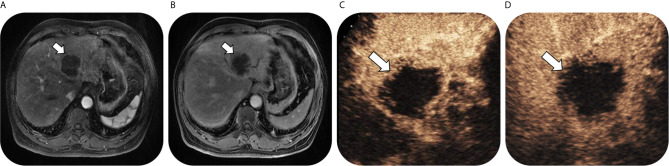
Images in a 63-year-old man with chronic hepatitis B virus infection and pathological confirmed intrahepatic cholangiocarcinoma lesion, which was correctly classified as LR-M both on CEUS and gadopentetate-enhanced MRI. T1-weighted image shows a 58-mm nodule in hepatic segment II/III/IV with rim arterial phase hyperenhancement (arrow) in (A) arterial phase followed by delayed central enhancement (arrow) in (B) portal phase. Contrast-enhanced US image shows a 68-mm nodule with rim arterial phase hyper-enhancement (arrow) in (C) arterial phase (timer, 00:22) followed by marked washout (arrow) visible in (D) portal phase (timer, 01:49).
